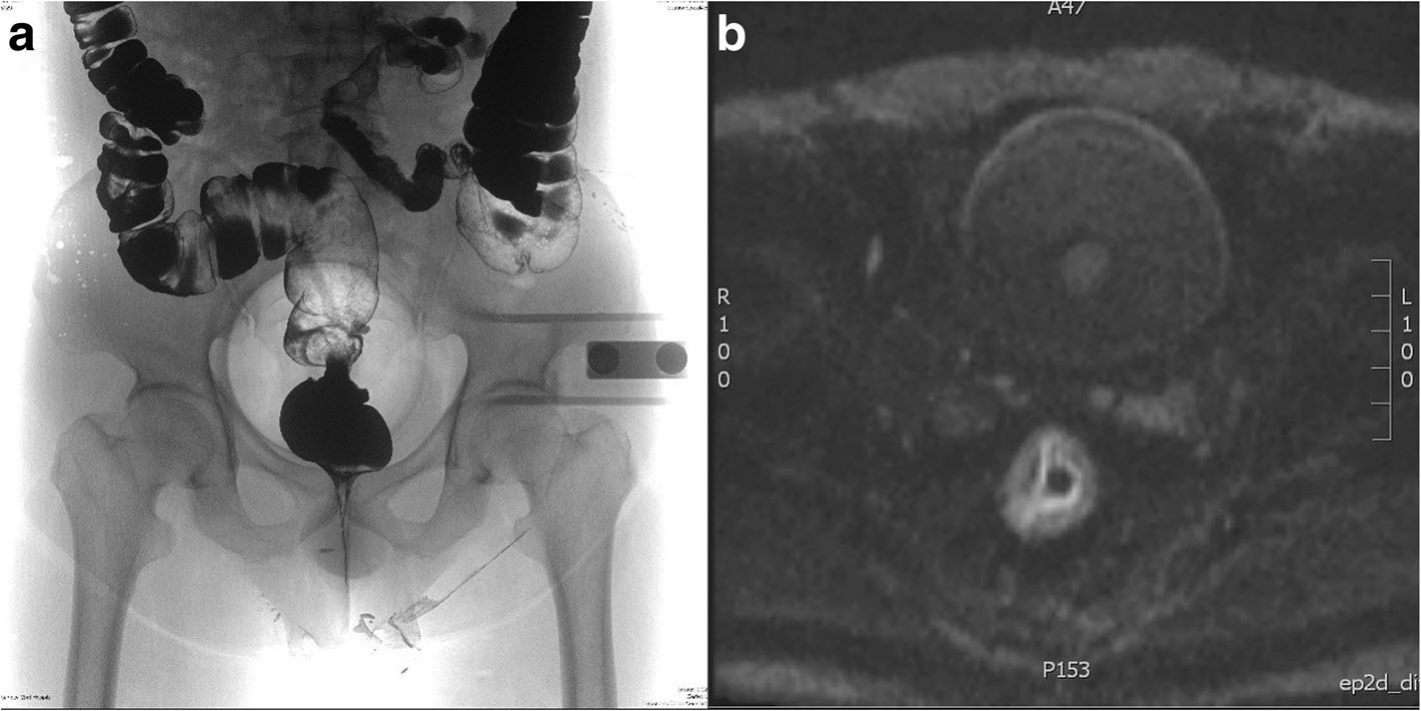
a The double-contrast barium enema showed an irregular rectal stenosis, nodulous filling defect and stiffness of involved rectal wall with destruction of mucosa. b The magnetic resonance imaging scan revealed the tumor invaded through the muscularis propria and the serosa was suspiciously involved, while at least 2 enlarged perirectal lymph node was found
